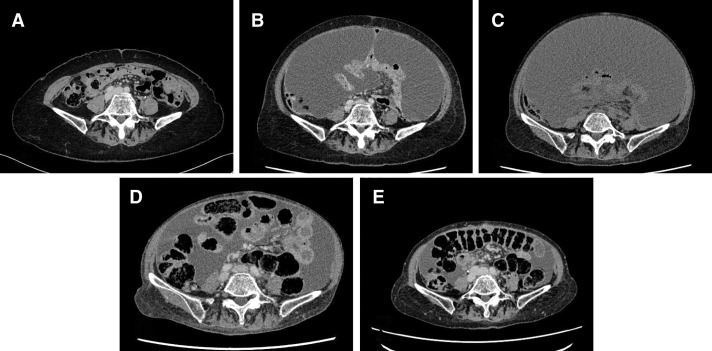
Serial axial CT images showing changes in ascites during the course of treatment. (A) During continued treatment with fulvestrant. (B) At initiation of S-1. (C) Prior to initiation of T-DXd. (D) After 4 cycles of T-DXd. (E) During maintenance phase of T-DXd therapy. The initiation of T-DXd therapy resulted in a reduction in ascites, which was sustained over time and contributed to an improvement in the patient’s ADL. ADL, activities of daily living; T-DXd, trastuzumab deruxtecan
